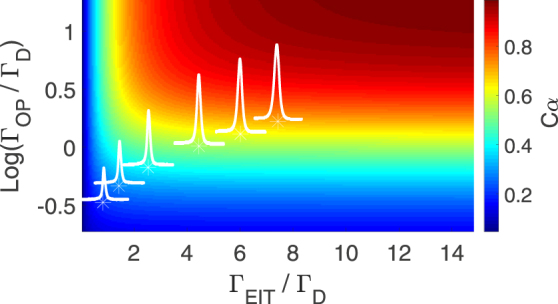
The calibration factor *C*_*α*_ for a Doppler-broadened medium is a function of optical pumping linewidth Γ_OP_ and EIT linewidth Γ_EIT_. The EIT peak transmission $$T(0)=\exp [-\mathrm{(2}\alpha \gamma {\rm{\Gamma }}/{{\rm{\Omega }}}_{c}^{2}){C}_{\alpha }]$$ with the effective OD of *αC*_*α*_. The six EIT spectra in Fig. 2(c) are also shown here with the corresponding Γ_OP_/Γ_D_ and Γ_EIT_/Γ_D_.
